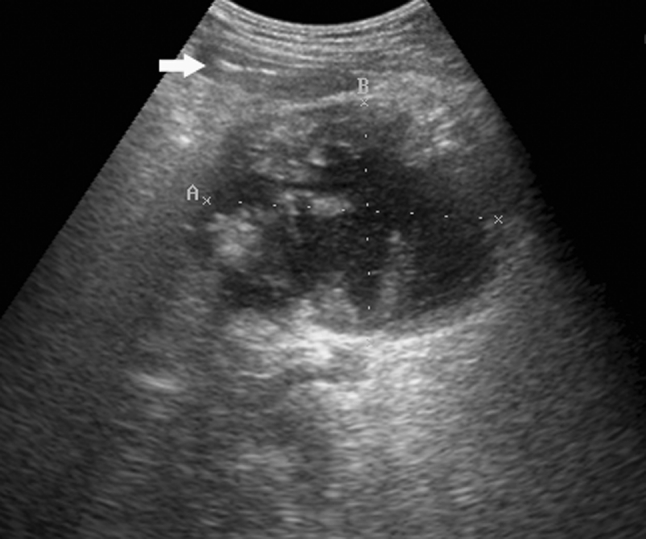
Transverse ultrasound scan demonstrating a fluid–solid collection filled with the necrotic debris (walled-off peripancreatic necrosis), which extends along the descending colon (*arrow*)
